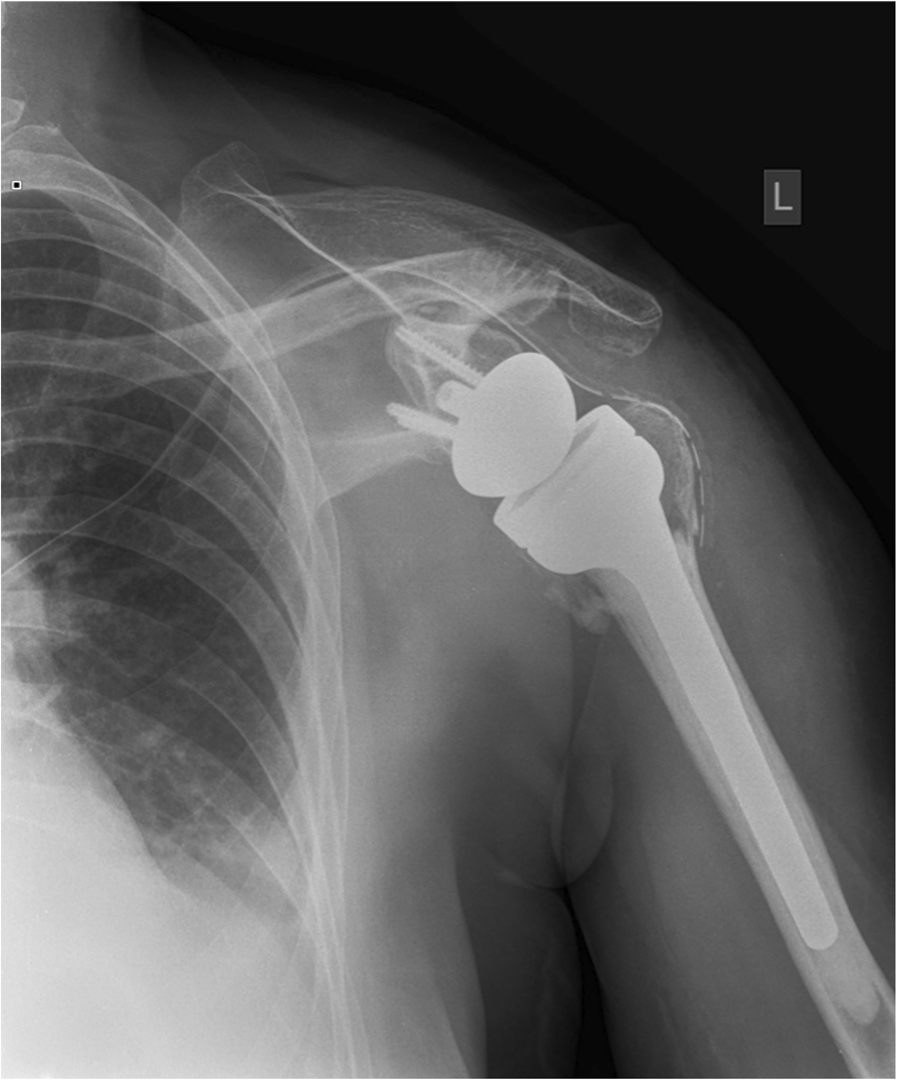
X-ray after implantation of reverse shoulder arthroplasty with refixation of greater tuberosity (a.-p.-view).
